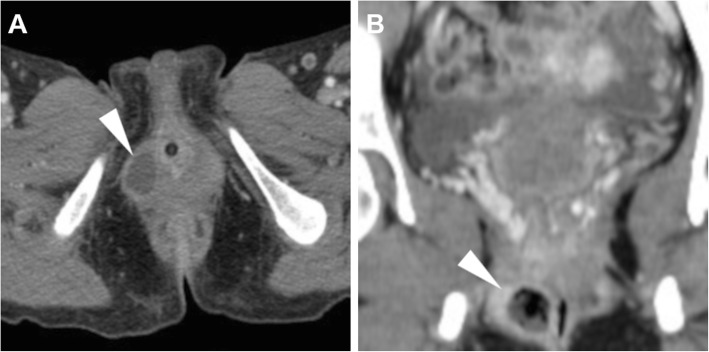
Two cases of vulvar abscesses (arrowheads) which required surgical incision. a Axial CT image of a small ovoid fluid collection with peripheral enhancement in the left-sided major labia. b Coronal CT image of an abscess with mixed fluid and gaseous content secondary to Bartholin gland infection
